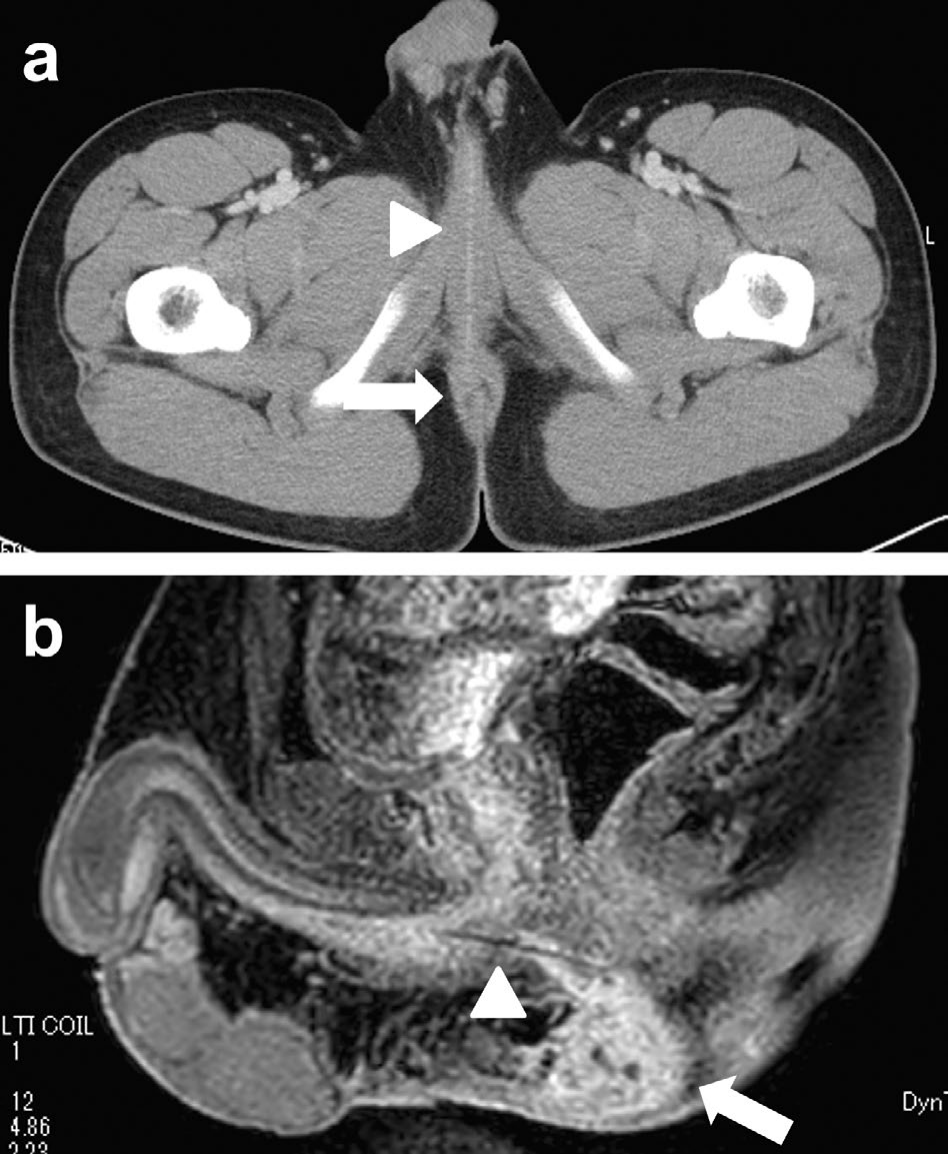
Computed tomography reveals the presence of a perianal abscess (arrow) and a perineal linear high‐density structure running anterior to posterior (arrow head) (a). Magnetic resonance imaging demonstrates a linear low‐intensity structure (arrow head), extending from the bulbar urethra to the perianal abscess (arrow) (b).
